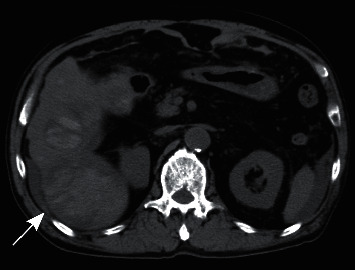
Nonenhanced computed tomography image. Perilesional blood was also observed near the hepatic tumor in the right lobe of the liver that was considered to indicate rupture of the HCC (arrow).
